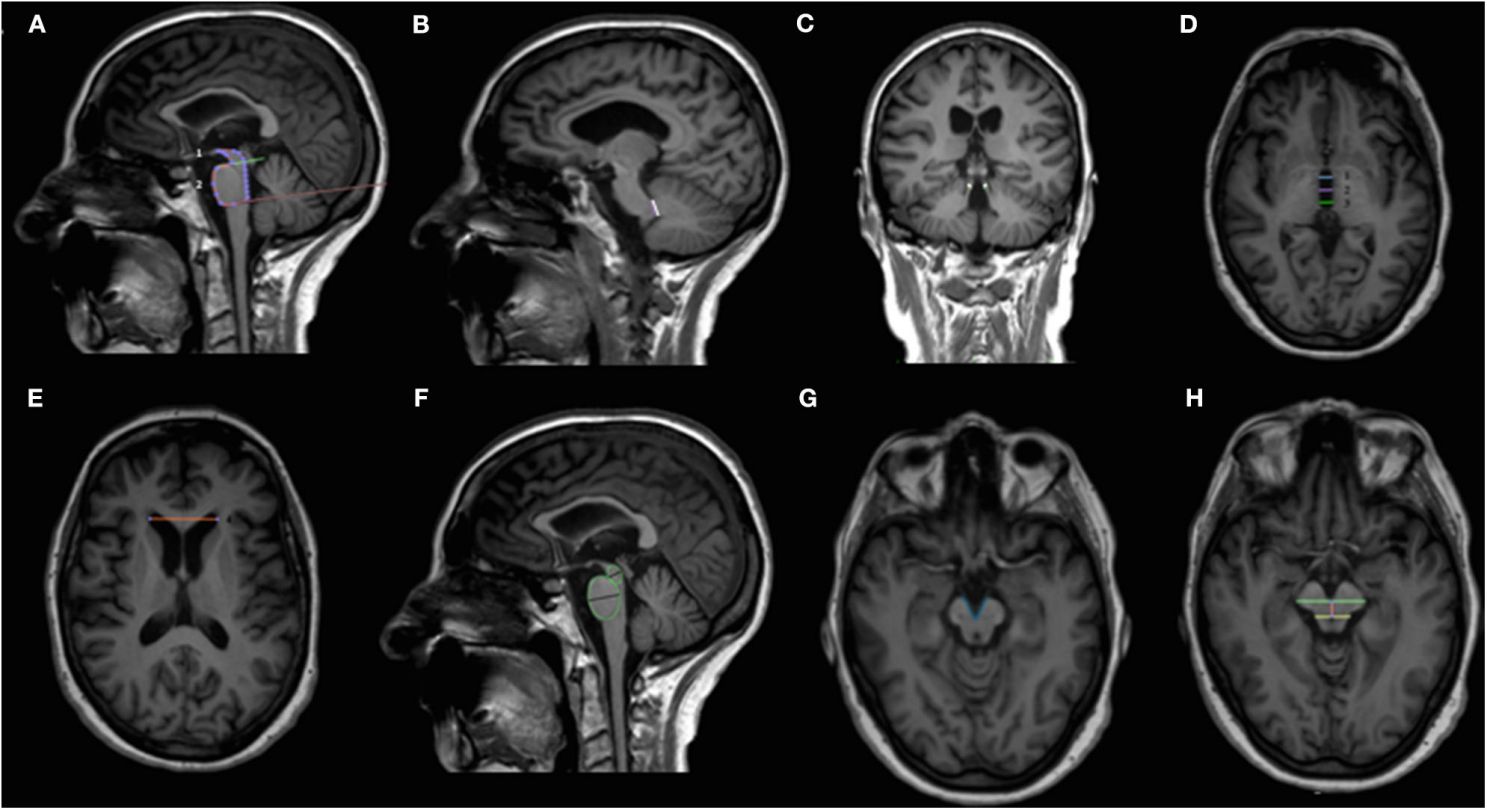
Overview of the midbrain-based measures computed. Sagittal (A,B,F), coronal (C) and axial (D,E,G,H) T1-weighted volumetric MR images. Midsagittal midbrain area (1) and midsagittal pons area (2) used for calculating the pons-to-midbrain area ratio (P/M) are shown in (A). Middle and superior cerebellar peduncles used to compute middle cerebellar peduncles to superior cerebellar peduncles ratio (MCP/SCP) are shown in (B) and (C), respectively. Third ventricle measurements at the level of anterior and posterior commissures [third ventricle width derives from the mean of the anterior (1), medium (2), and posterior lines (3)] and frontal horns width are shown in (D) and (E), respectively. MRPI is calculated with the following formula: (P/M) × (MCP/SCP); MRPI 2.0 is calculated with the following formula: MRPI × (third ventricle width/frontal horns width); P/M 2.0 is calculated with the following formula: (P/M) × (third ventricle width/frontal horns width). Midbrain and pons diameters obtained from midsagittal elliptical regions of interests are shown in (F). Minor axes were used to calculate the pons-to-midbrain diameter ratio. The interpeduncular angle, calculated on a plane parallel to anterior commissure-posterior commissure line and right below the mammillary bodies, is shown in (G). The length of midbrain tegmentum, measured as the distance between the interpeduncular fossa and the center of the aqueduct at the level of mammillary bodies, on a plane parallel to the anterior commissure-posterior commissure line is shown in (H). MCP/SCP, middle cerebellar peduncles to superior cerebellar peduncles ratio; MR, magnetic resonance; MRPI, MR Parkinsonism index; MRPI 2.0, MR Parkinsonism index 2.0 version; P/M, pons-to-midbrain area ratio; P/M 2.0, pons-to-midbrain area ratio 2.0 version.
